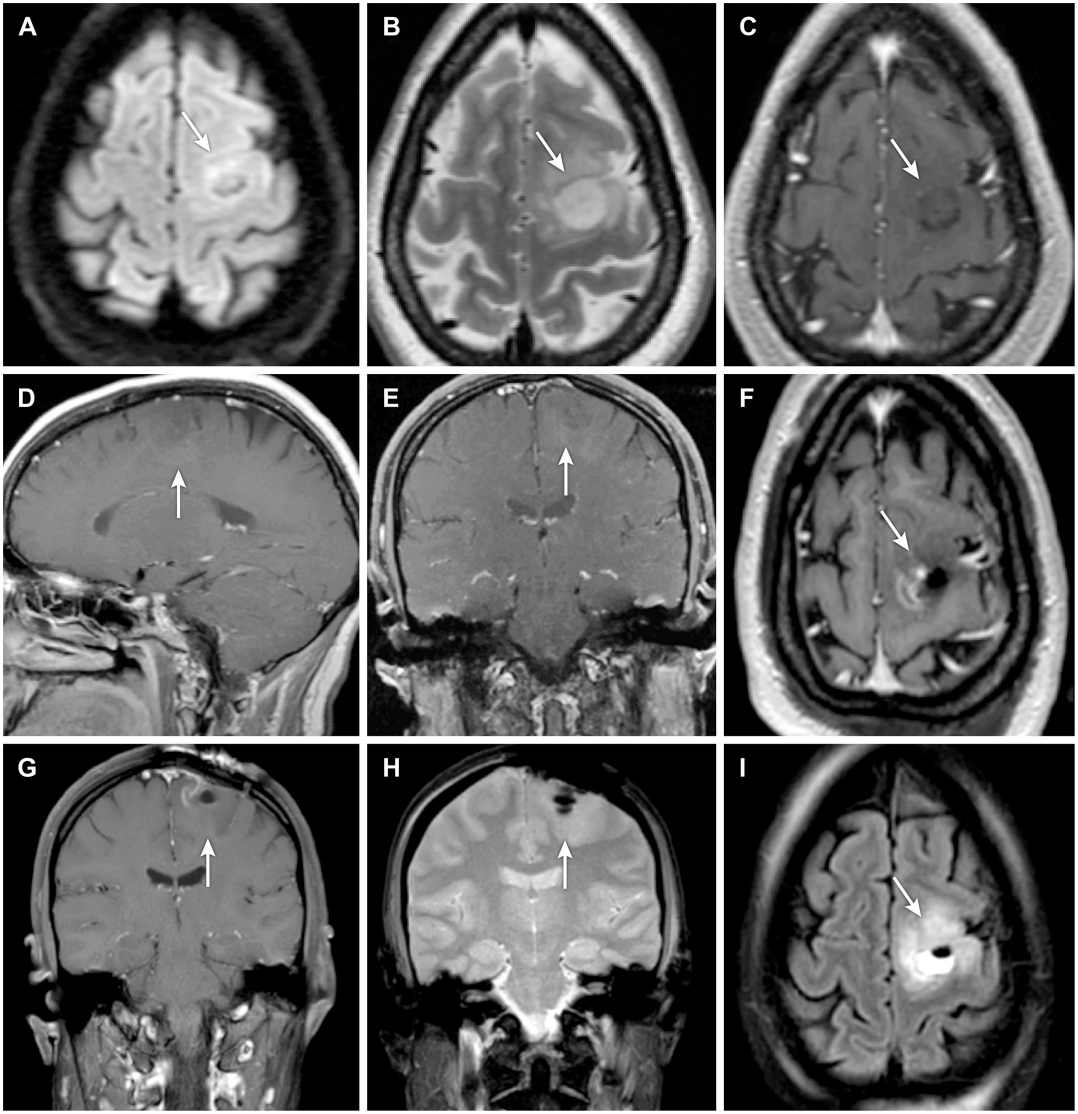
(A–E) Preoperative and (F–I) postoperative magnetic resonance imaging (MRI) of a patient with a nonenhancing lesion (A–I, *arrows*) in the left precentral gyrus. Preoperative MRIs shown are (A) axial diffusion-weighted, (B) axial T2-weighted, (C) axial T1-weighted, (D) sagittal T1-weighted with contrast, and (E) coronal T1-weighted with contrast. Postoperative MRIs shown are (F) axial T1-weighted with contrast, (G) coronal T1-weighted with contrast, (H) coronal diffusion-weighted, and (I) axial diffusion-weighted. Used with permission from Barrow Neurological Institute, Phoenix, Arizona.
